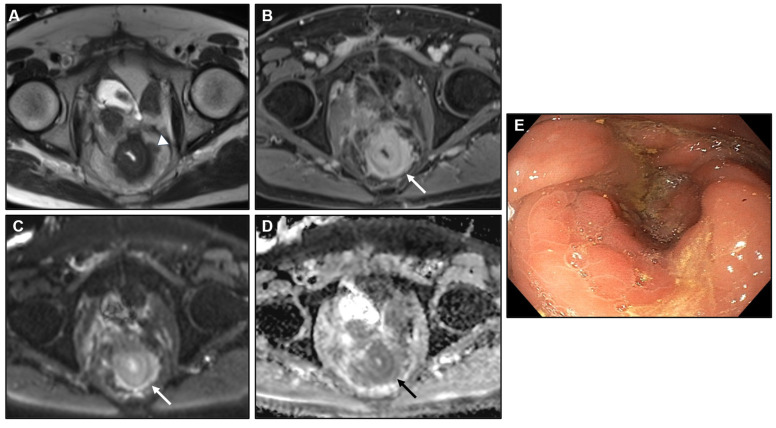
Extramural tumour component markedly hypointense on T2WI. (A) MR image: Axial T2WI. (B) MR image: Axial CE-GRE-T1WI. (C) MR image: DWI, (D) MR image: ADC. (E) Colonoscopy. Patient with bladder adenocarcinoma previously treated with surgery and chemotherapy. MRI showed an irregular contour with a strikingly hypointense extramural tumor component on T2WI (arrowhead in (A)), reflecting intense desmoplastic reaction. A concentric ring pattern of the rectum was evident, more pronounced on DWI (arrows in (C,D)), along with a malignant target sign (arrow in (B)). Colonoscopy revealed extrinsic rectal infiltration causing luminal occlusion, suspected to represent linitis plastica-like metastasis from bladder adenocarcinoma; however, biopsy results were negative. The patient subsequently underwent colostomy, during which peritoneal metastases were identified. The patient died shortly thereafter, and the diagnosis could not be definitively confirmed.
